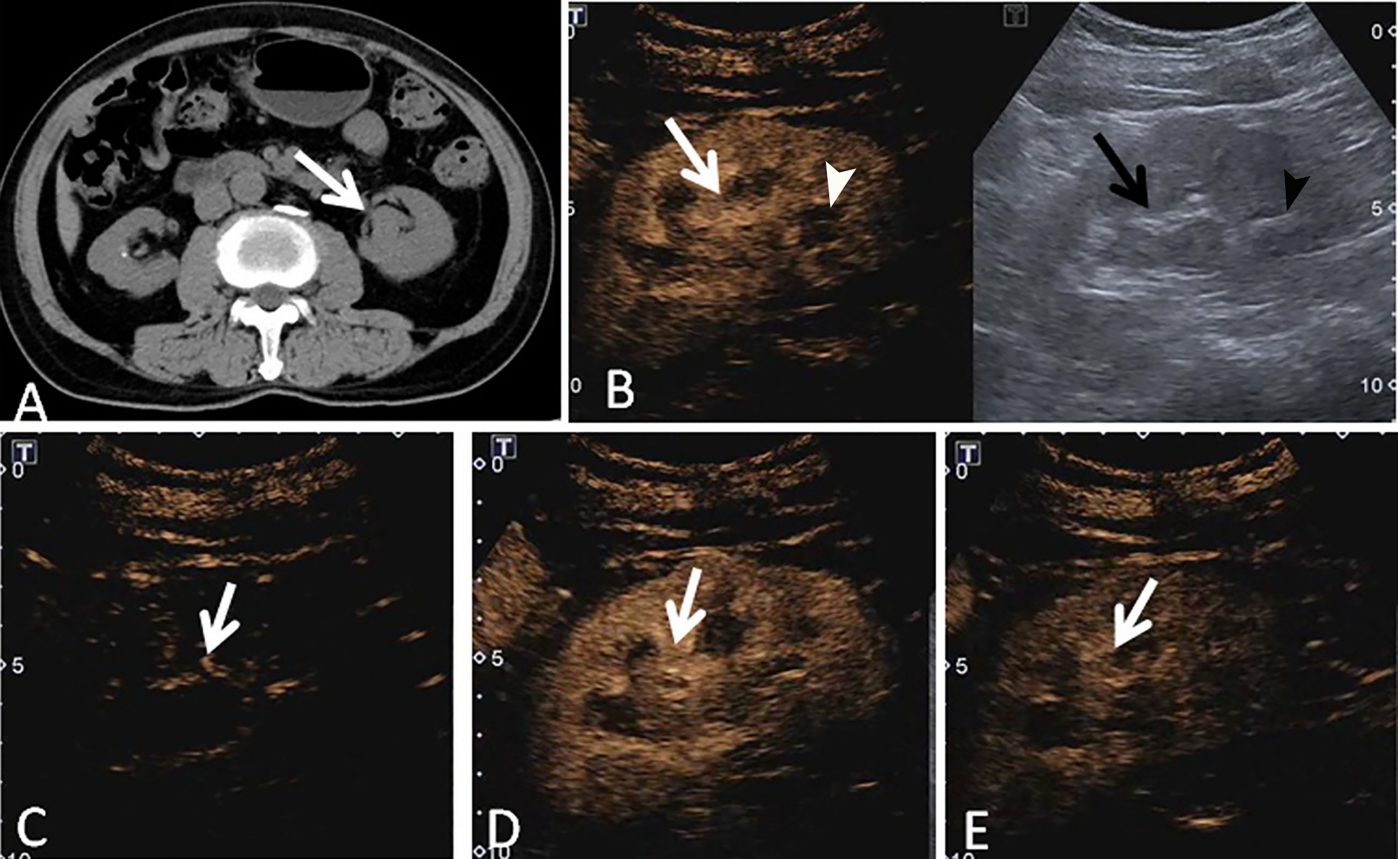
A 73-year-old man had a UCRP in the left kidney with the size of 3.4 × 1.9 cm. (A) Non-enhanced CT scan showed that there may be blood clots in the renal pelvis (white arrow). (B) According to the position displayed on CT, the simultaneous display mode of CUS (right) and CEUS (left) was performed. No obvious lesion (black arrow) was detected on CUS, but it showed a slight separation of the renal pelvis and a stone (black arrowhead) in the lower part of the renal pelvis. On CEUS, a lesion with enhancement was observed (white arrow), and the stone showed no enhancement (white arrowhead). (C) The contrast agent entered the lesion more slowly than that of the adjacent renal cortex (white arrow). (D) The tumor showed heterogeneous enhancement, and the enhancement intensity of the tumor (white arrow) was similar to that of the adjacent renal cortex. (E) The contrast agent discharged from the mass quickly, and the tumor (white arrow) showed a fast-out pattern compared to the renal cortex.
